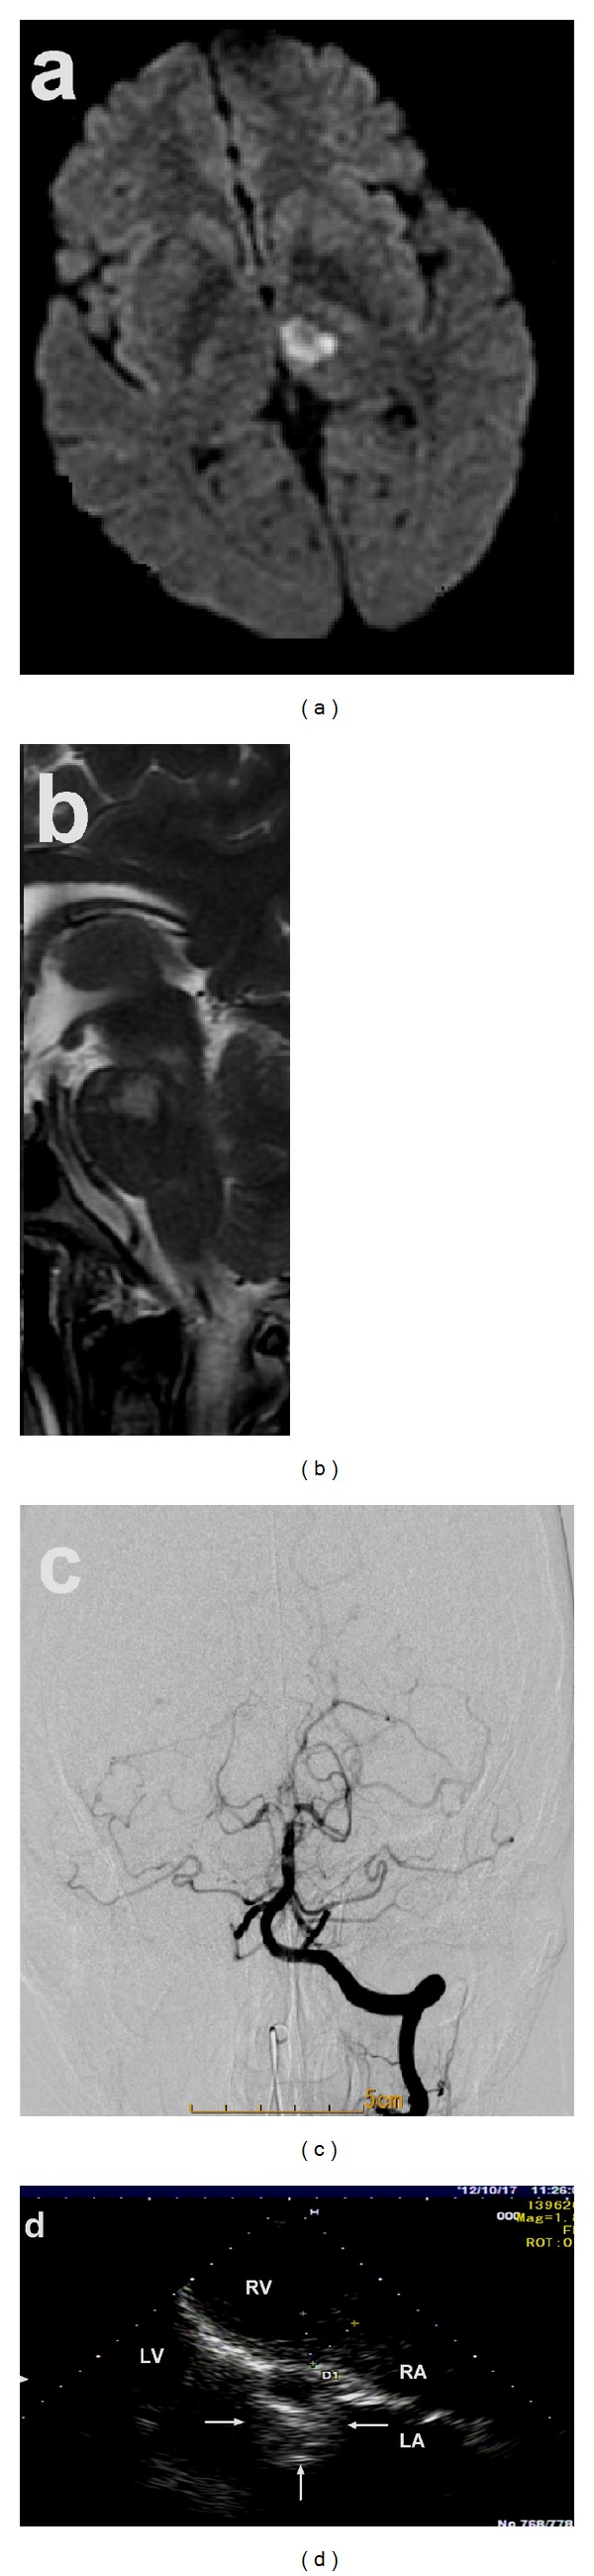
A patient presented with NMO-like attack. A 34-year-old woman with bilateral impaired vision, double vision, quadriplegia, dysuria, and internuclear ophthalmoplegia had normal brain CT results on 14 October 2012. Brain MRI showed multiple new lesions in brain. (a) MRI revealed abnormalities beside the third ventricle. (b) MRI revealed lesions in the pons. (c) DSA revealed occlusion of the top of the basilar artery. (d) Echocardiography showed a highly mobile mass attached to the fossa ovalis of the left atrium (arrow).
